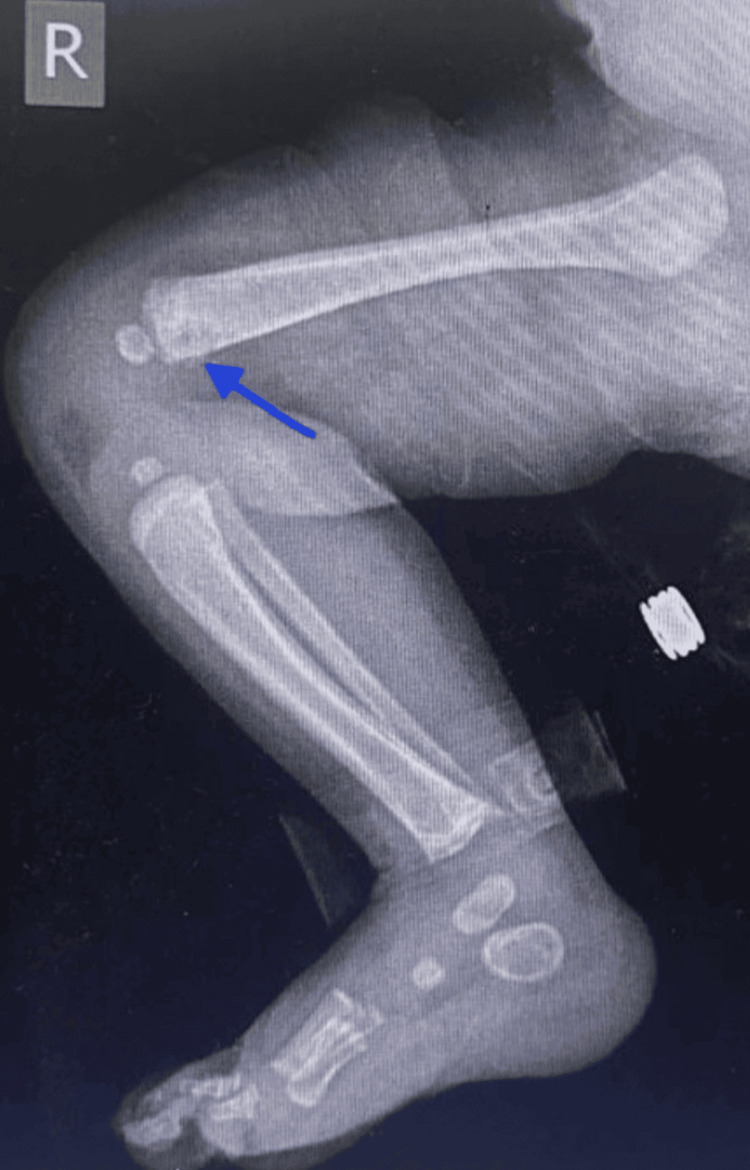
X-ray scan shows area of destruction in the distal shaft of the right femur.
